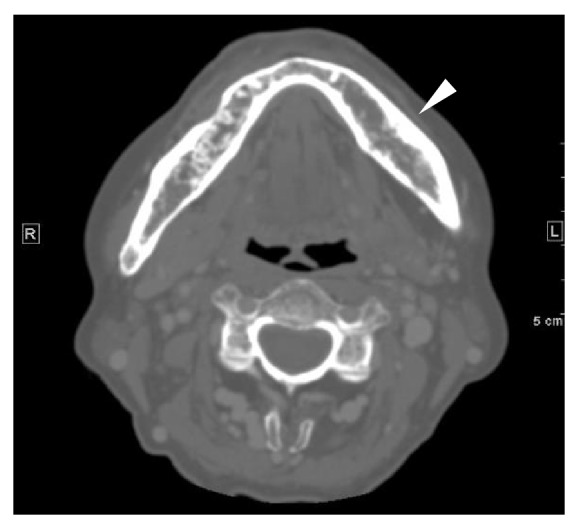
An enhanced CT scan indicated the mixture of acute and chronic osteomyelitis in the left mandible.
